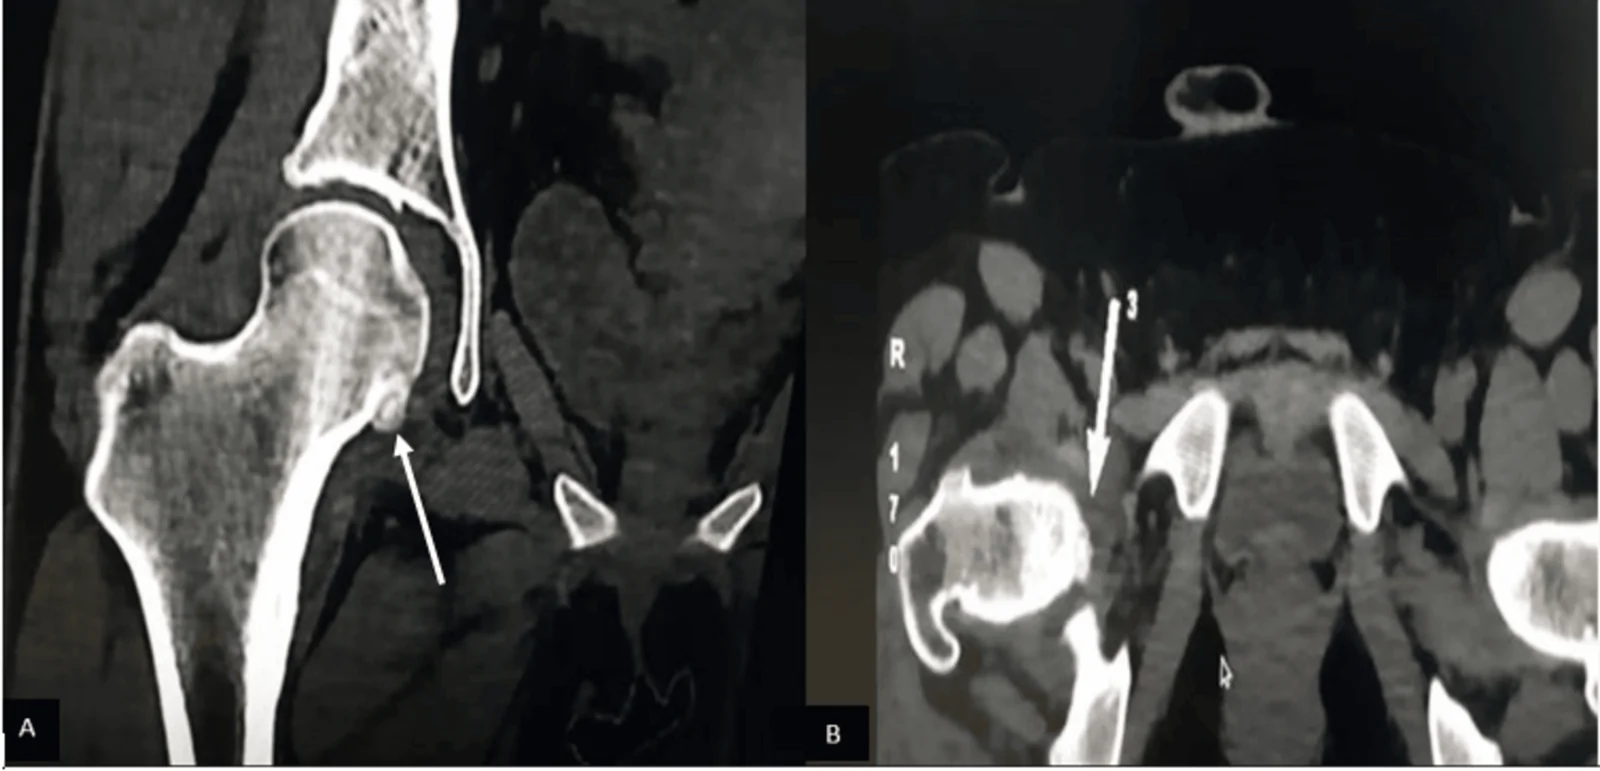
CT images of the right hip (A) Coronal CT image demonstrating a well-circumscribed nidus in the inferomedial subcapital region of the right femoral neck (arrow), surrounded by mild reactive sclerosis. (B) Axial CT image confirming the intra-articular nidus (arrow), consistent with osteoid osteoma CT: computed tomography
